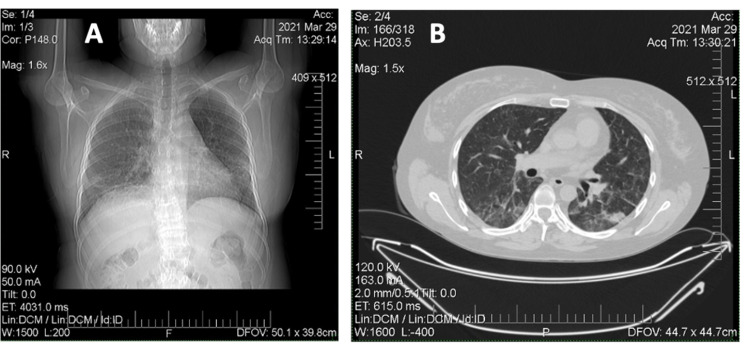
A, B: Multislice computed tomography of the chest (March 2021). A: Vertical tomography slices. B: Horizontal tomography slices. Pronounced thickening of the peribronchial interstitial lung parenchyma with coarse fibrous layers, predominantly located in the hilar regions and lower lobes of the lungs. The lumen of the subsegmental bronchi of the lower lobes is moderately widened - traction bronchiectasis is formed. Negative dynamics in the form of developing pulmonary fibrosis, the formation of traction bronchiectasis.
